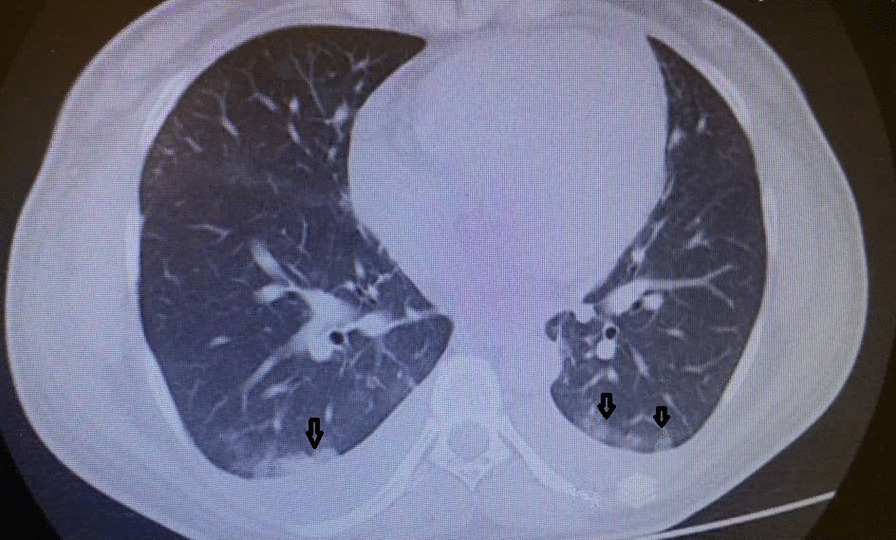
Chest computed tomography showing peripheral patchy and ground-glass opacities (arrows) in both lungs and pleural effusion
